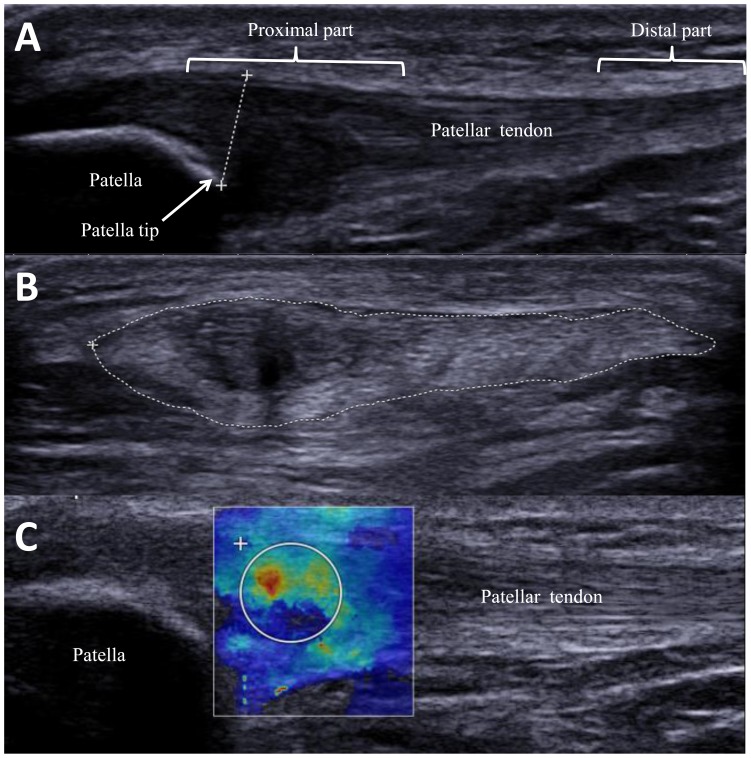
Sonography images of the patellar tendon (A) Thickness of the patellar tendon (dotted line) was measured from the superior border of the patellar tendon to the tip of the patellar. (B) Cross-sectional area of the patellar tendon was measured by tracing the outer margin of the patellar tendon (dotted circle) (C) Shear elastic modulus of the patellar tendon was quantified by the elastography. The white circle delineates the area of interest.
